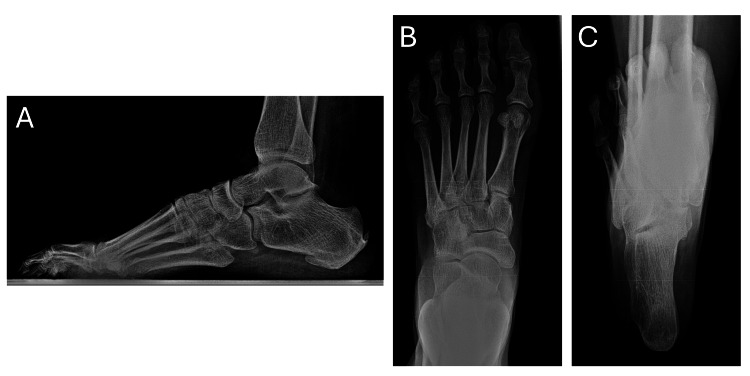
Initial consultation radiographs Pre-procedure left foot radiographs, including lateral, AP, and calcaneal axial views, taken in May 2014. (A) Lateral view reveals flexion contractures of digits 2-5. (B) AP view demonstrates decreased first metatarsal protrusion distance. (C) Calcaneal axial view shows an 8° calcaneal varus deformity.
